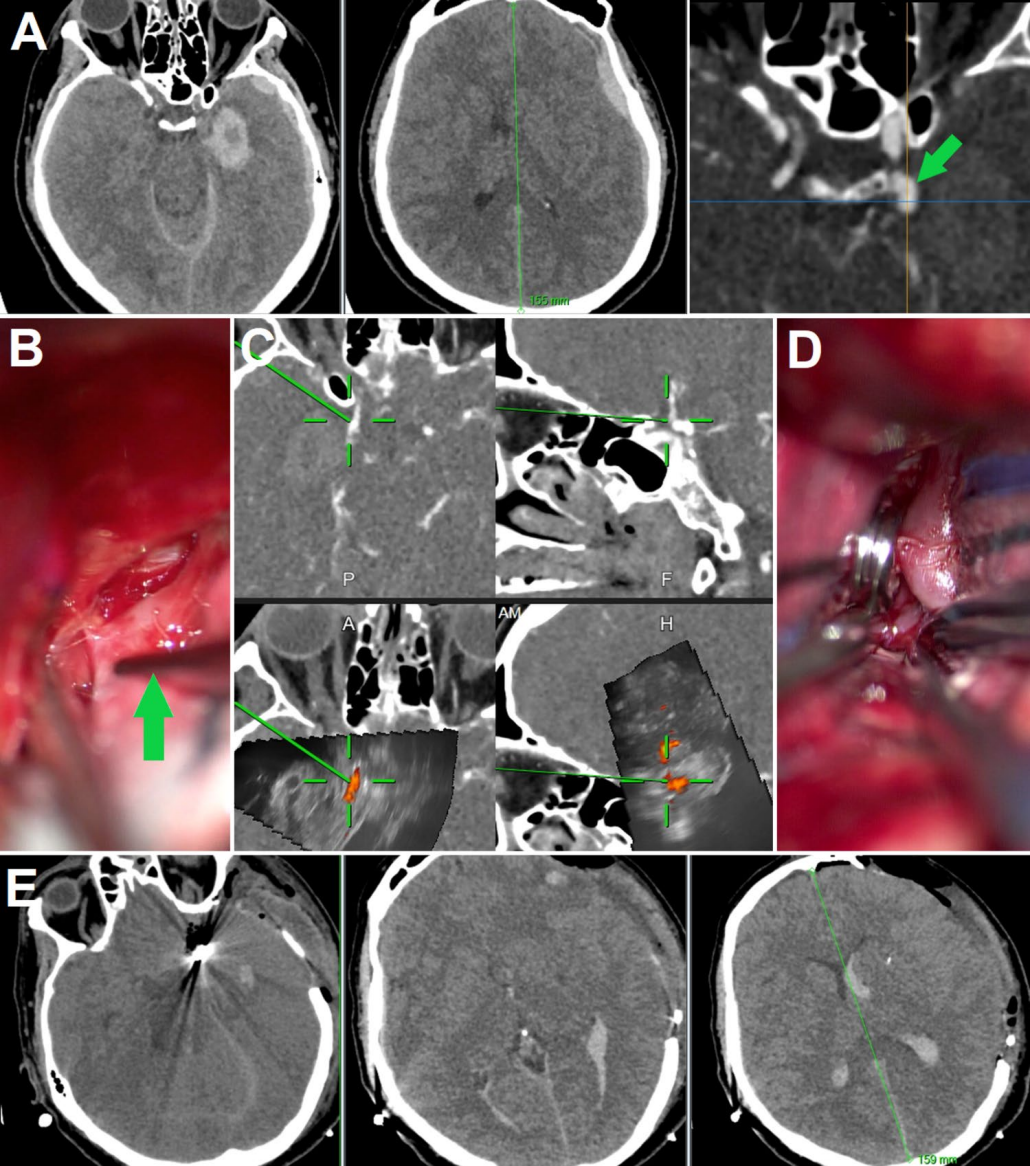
Ruptured posterior communicating artery aneurysm (case No. 21) A: CT and CT angiography images showing intracerebral and subdural hematoma after rupture of a left-sided Pcom aneurysm
